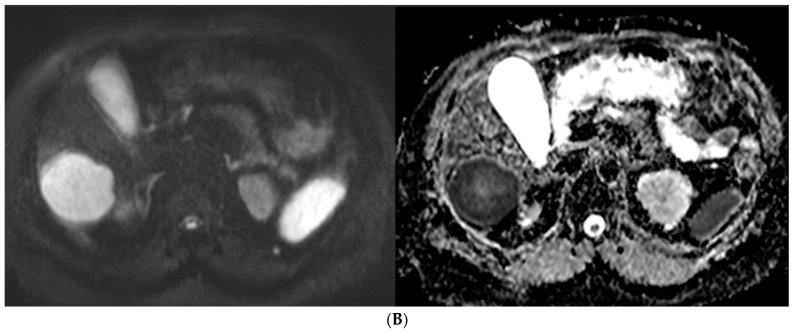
57-year-old man with well-differentiated G2 pancreatic neuroendocrine tumor referred for initial staging. (ADC = Apparent diffusion coefficient). (A) Axial ^68^Ga-DOTATATE PET (left) and fused PET/CT (right) images show intensely ^68^Ga-DOTATATE avid segment 6 deposit (green circle within metastasis represents region of interest, SUVmax, 45.0). (B) High signal intensity is seen on diffusion-weighted MR image with b value = 600 s/mm^2^ (left), and corresponding image on ADC map shows marked hypointensity (right).
